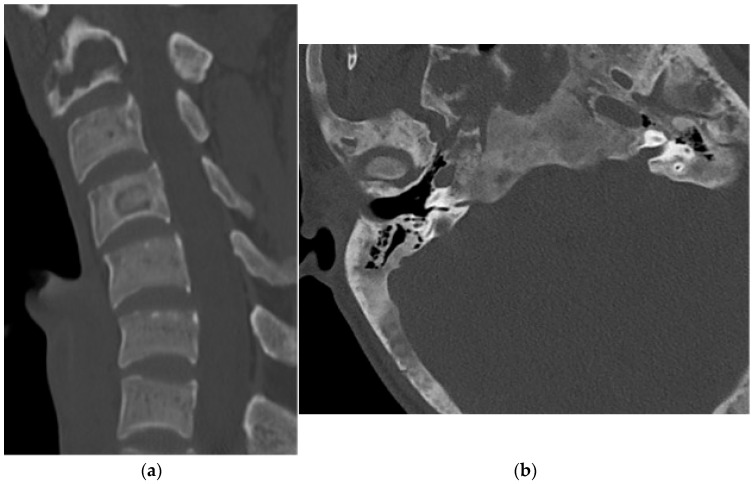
Fibrous dysplasia. (a) Sagittal CT bone window, “ground glass” lesion with peripheral lucency in the center of the C4 vertebral body. A lytic lesion with peripheral sclerosis is partially included in the C2 vertebral body. (b) Axial CT bone window through the skull base, extensive ground glass, and expansion in the calvarium at the skull base in a patient with McCune Albright syndrome.
